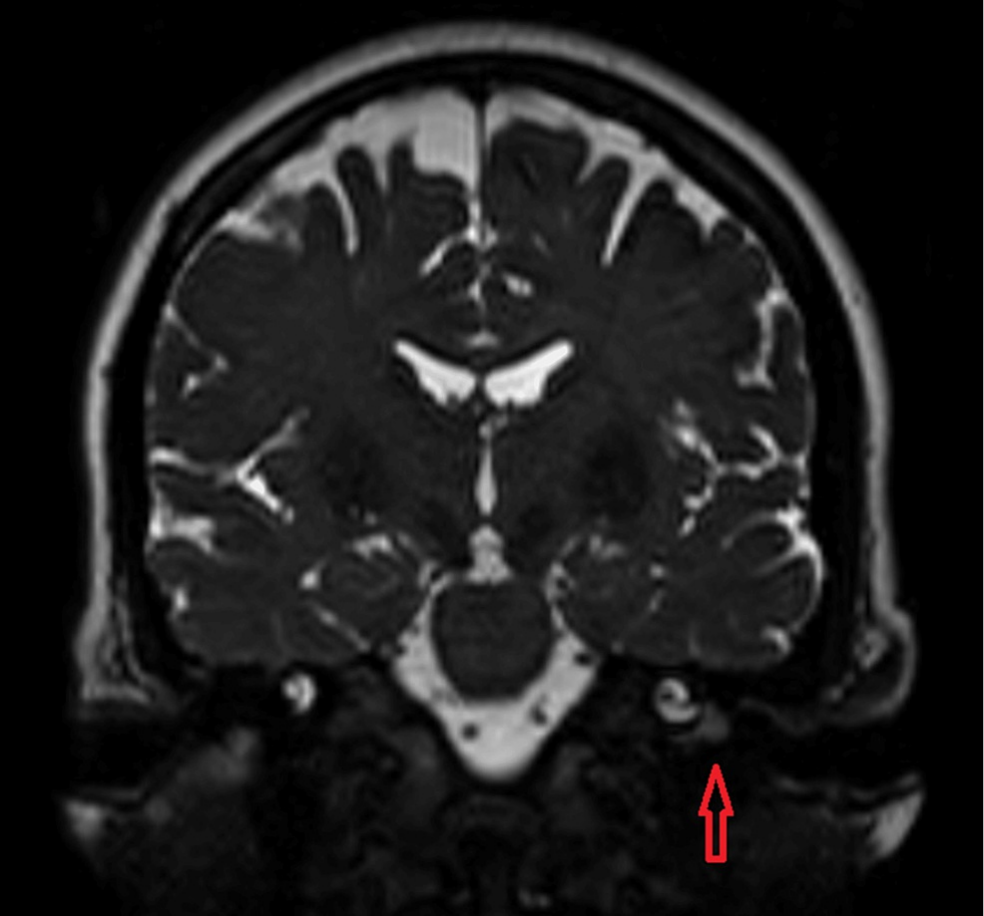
Coronal MRI T2 demonstrating an enhancing mass on the left cochlear promontory MRI: magnetic resonance imaging
